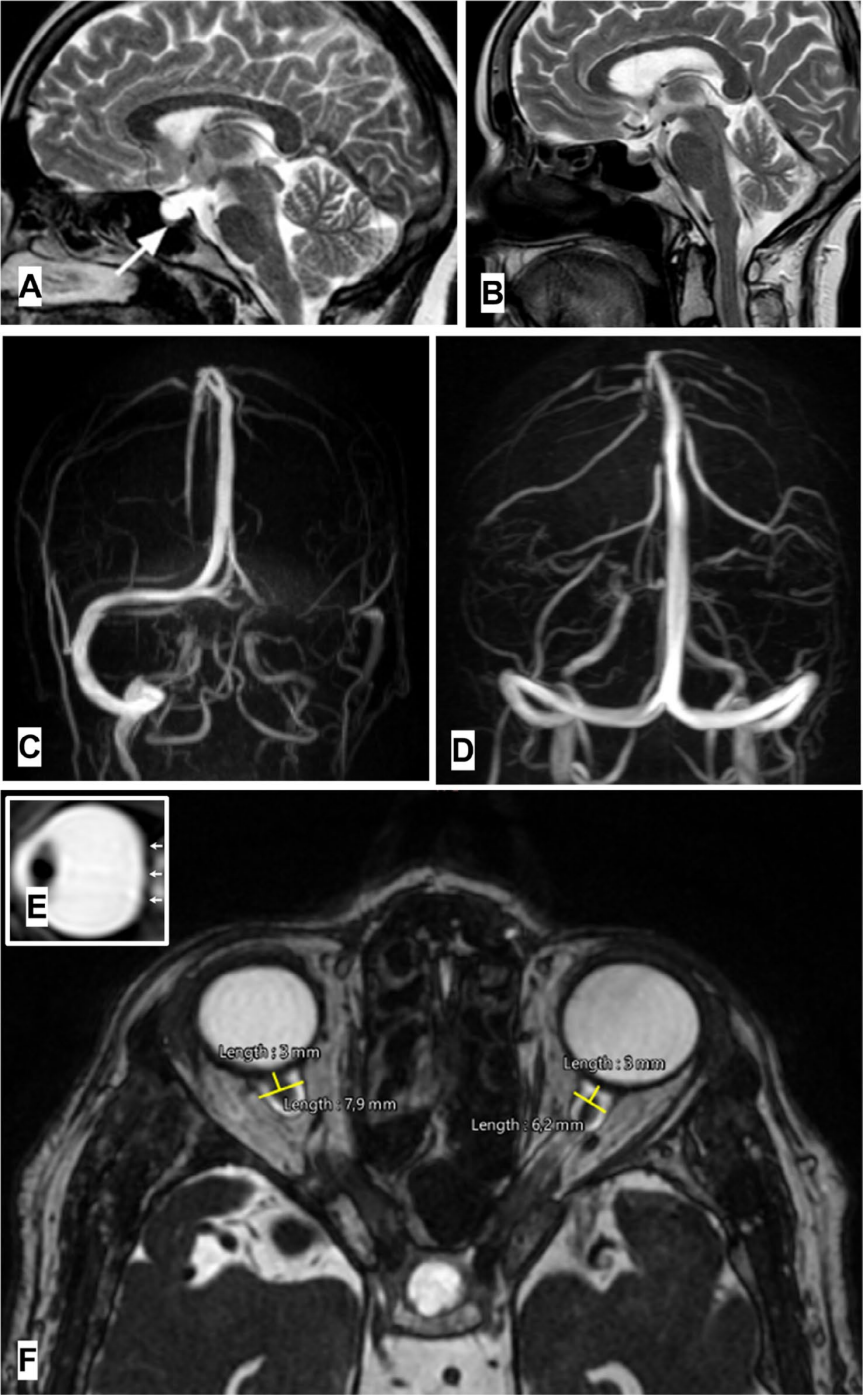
Indirect MRI sign of IIH: Empty sella (A) versus normal sella (B); dural sinus stenosis (C) versus normal dural sinuses (D); bulb flattening (E); enlargement of optic nerve sheaths (F).
